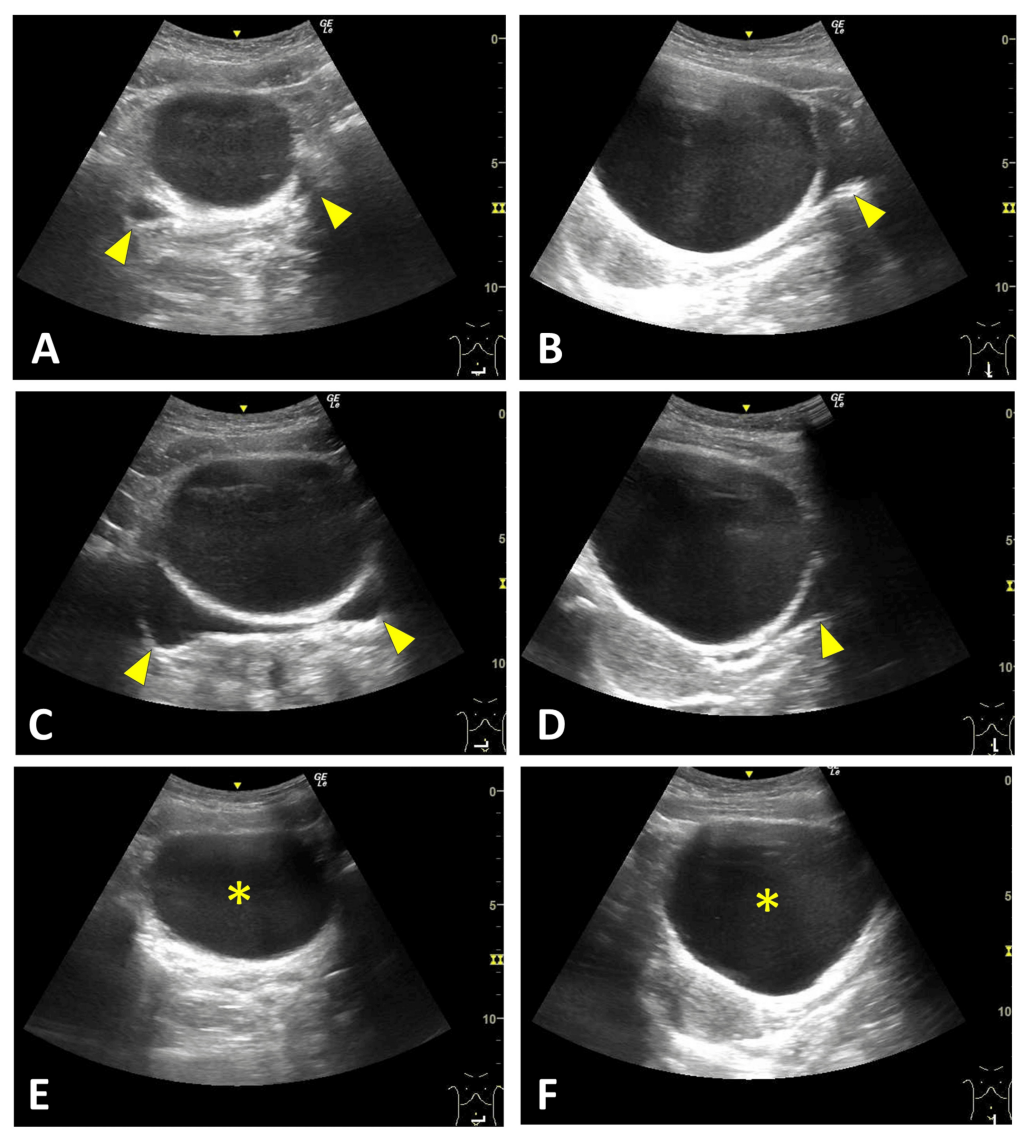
Cystic Pelvic Mass Mimicking the Urinary Bladder on POCUS Initial POCUS demonstrated an anechoic area in the transverse (A) and longitudinal (B) views, which was initially interpreted as ascites (arrowheads). Repeat prevoid scans showed an enlarged anechoic area (C, D; arrowheads). Subsequent postvoid scans confirmed that the region initially presumed to be the bladder was actually a cystic mass (*) (E, F). POCUS: point-of-care ultrasound
